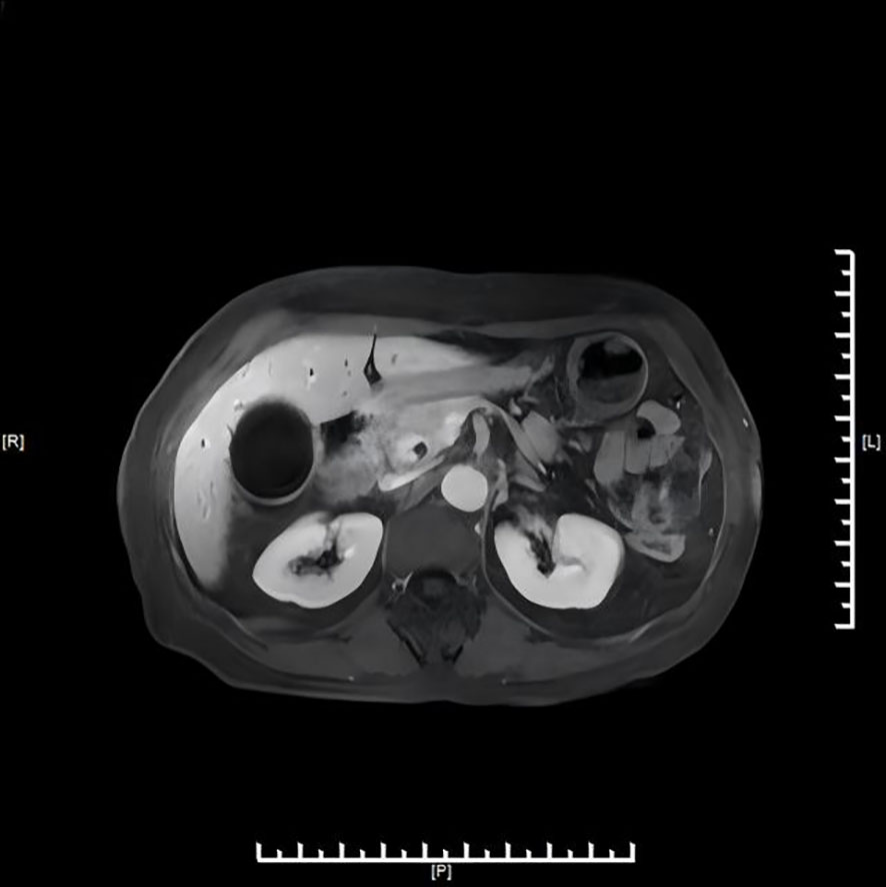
MRI/MRCP demonstrating low cystic duct insertion and irregular thickening at the cystic duct–common hepatic duct junction.
